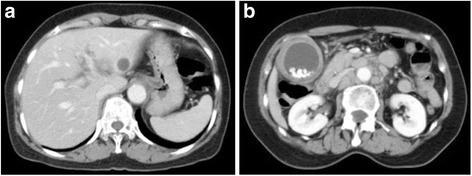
a An abdominal CT scan image shows dilatation of intrahepatic biliary duct. No mass lesions are seen in the liver. b A CT scan image shows multiple para-aortic lymph nodes swelling and multiple gallbladder stones with gallbladder wall thickness
